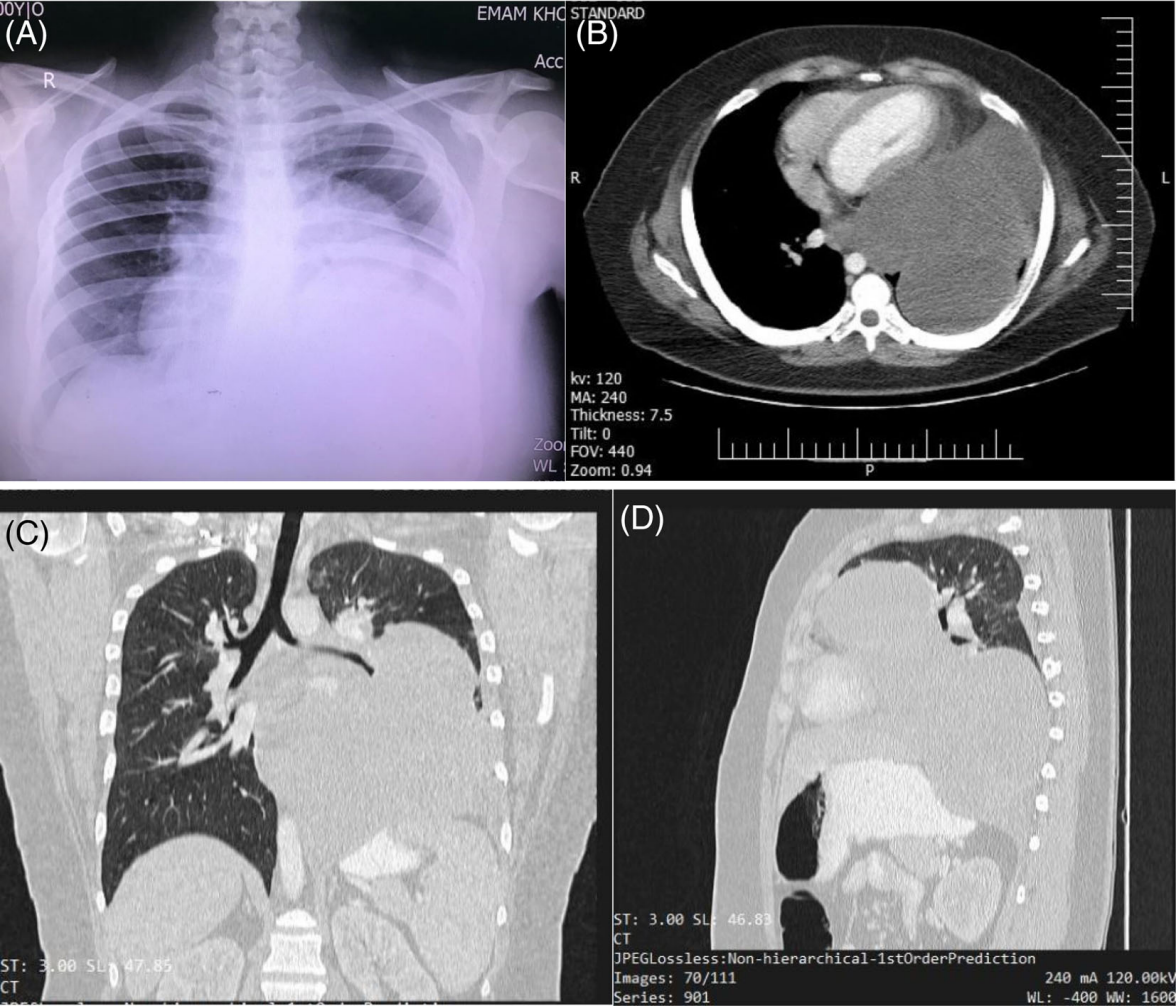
Huge lobulated mass in left hemithorax with a right‐sided shift of mediastinum. (A) chest X‐ray, (B) axial view of CT scan, (C) coronal view of CT scan, (D) sagittal view of CT scan
